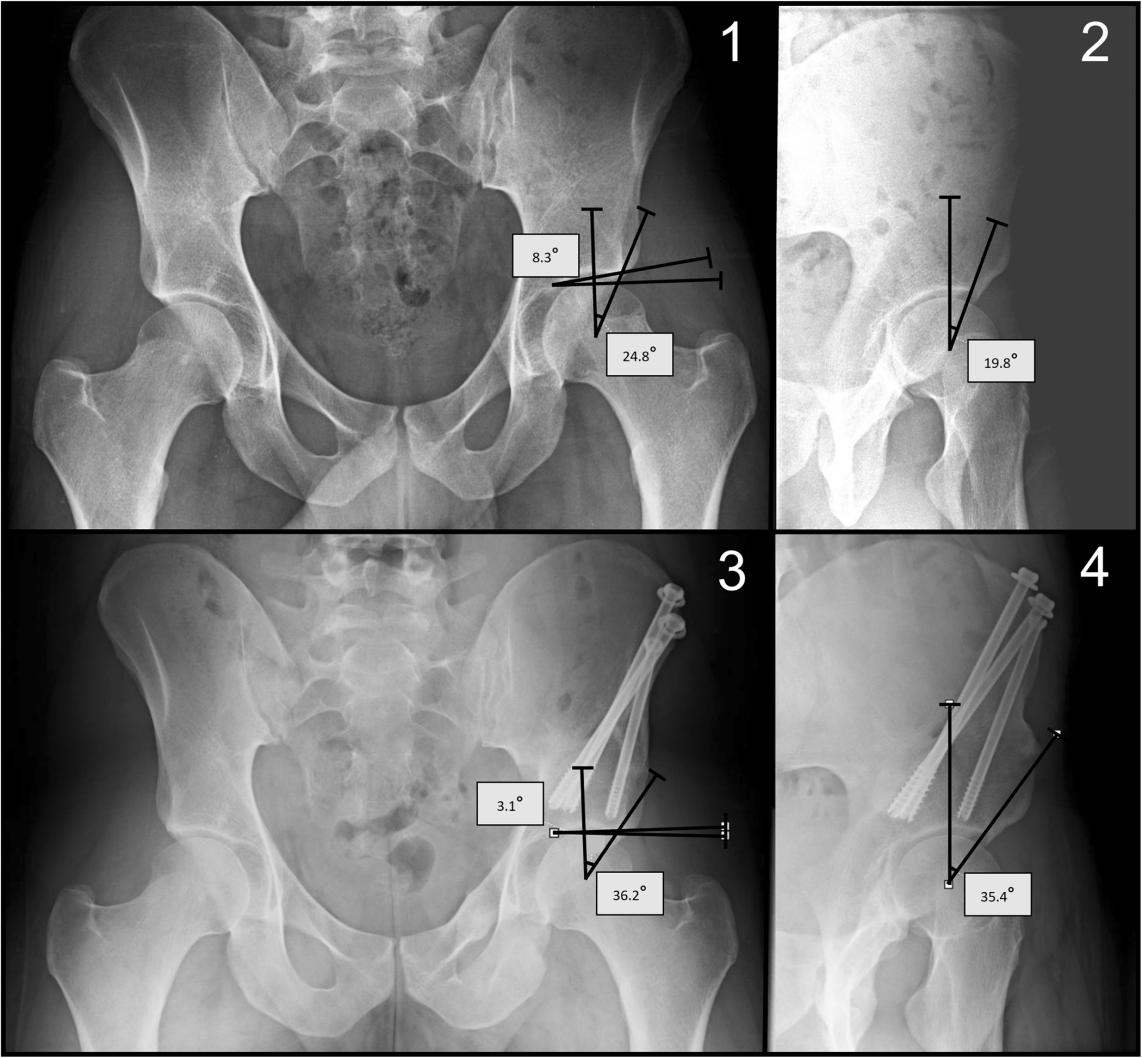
Examples of radiographical measurements. 1 and 3: Anterior‐posterior view of the pelvis, measurement of the “lateral center‐edge angle” (LCE angle) as well as the “acetabular roof angle” (HTE angle). 1 shows the preoperative measurement, 3 the postoperative measurement. 2 and 4: Faux‐profile projection with measurement of the “vertical‐center‐anterior angle” (VCA angle). 2 shows the preoperative measurement, 4 shows the postoperative measurement.
